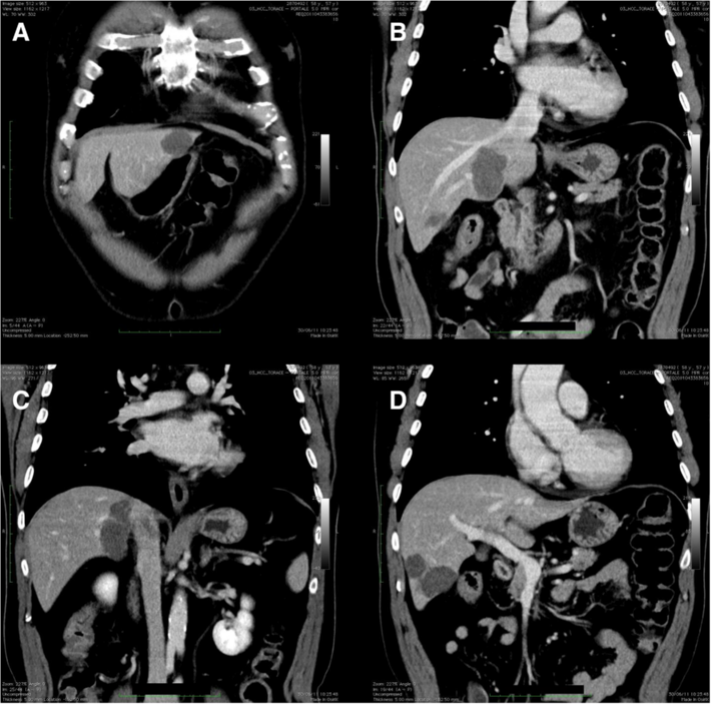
A CT scan demonstrated advanced bilobar disease. (A) Cancer nodule in the left lobe and (B, C, D) multiple gross deposits in the right lobe of the liver. The tumor also infiltrates S1. CT, computed tomography.
